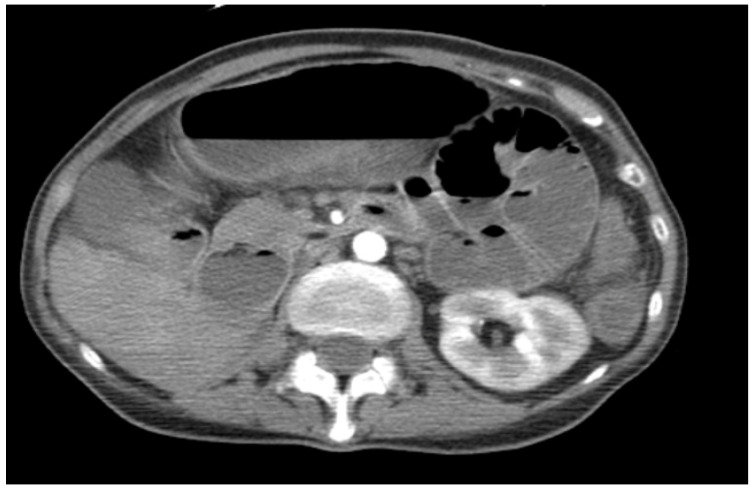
Abdominal CT showing ileocecal wall thickening with small bowel distension and stasis, perienteric fat stranding, and hepatomegaly; other abdominal organs appear normal.
